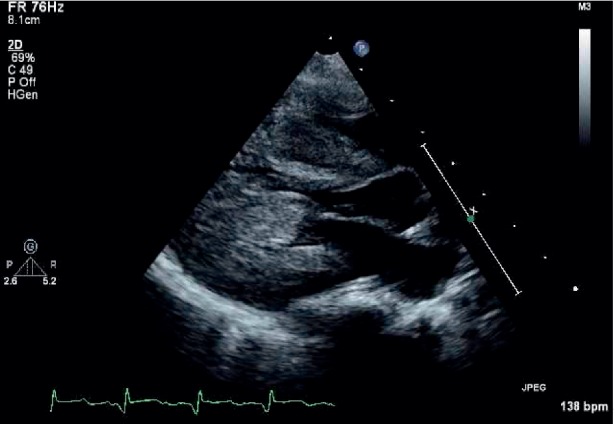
Parasternal long axis view of the patient's left ventricle showing marked concentric hypertrophy.
